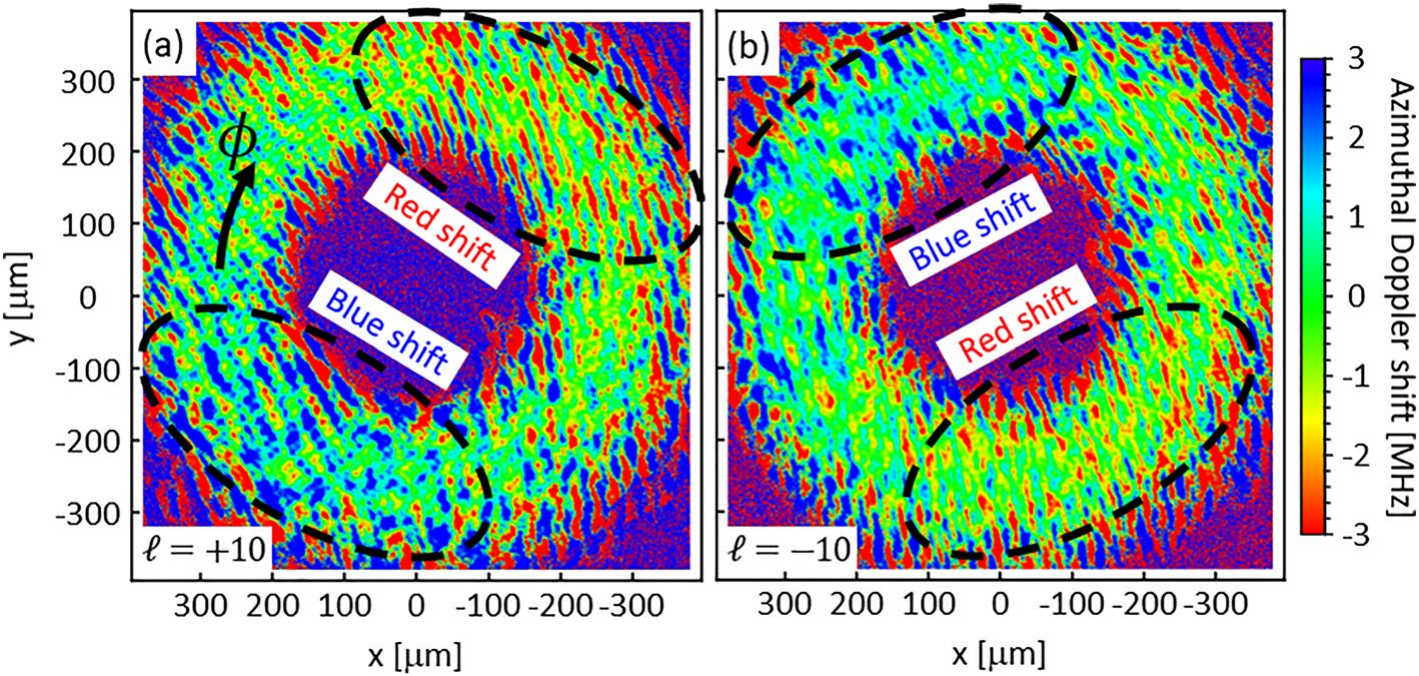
The azimuthal Doppler shift distribution observed by OVLAS. The distribution is rotated (a) clockwise for positive topological charge, (b) counterclockwise for negative topological charge.
